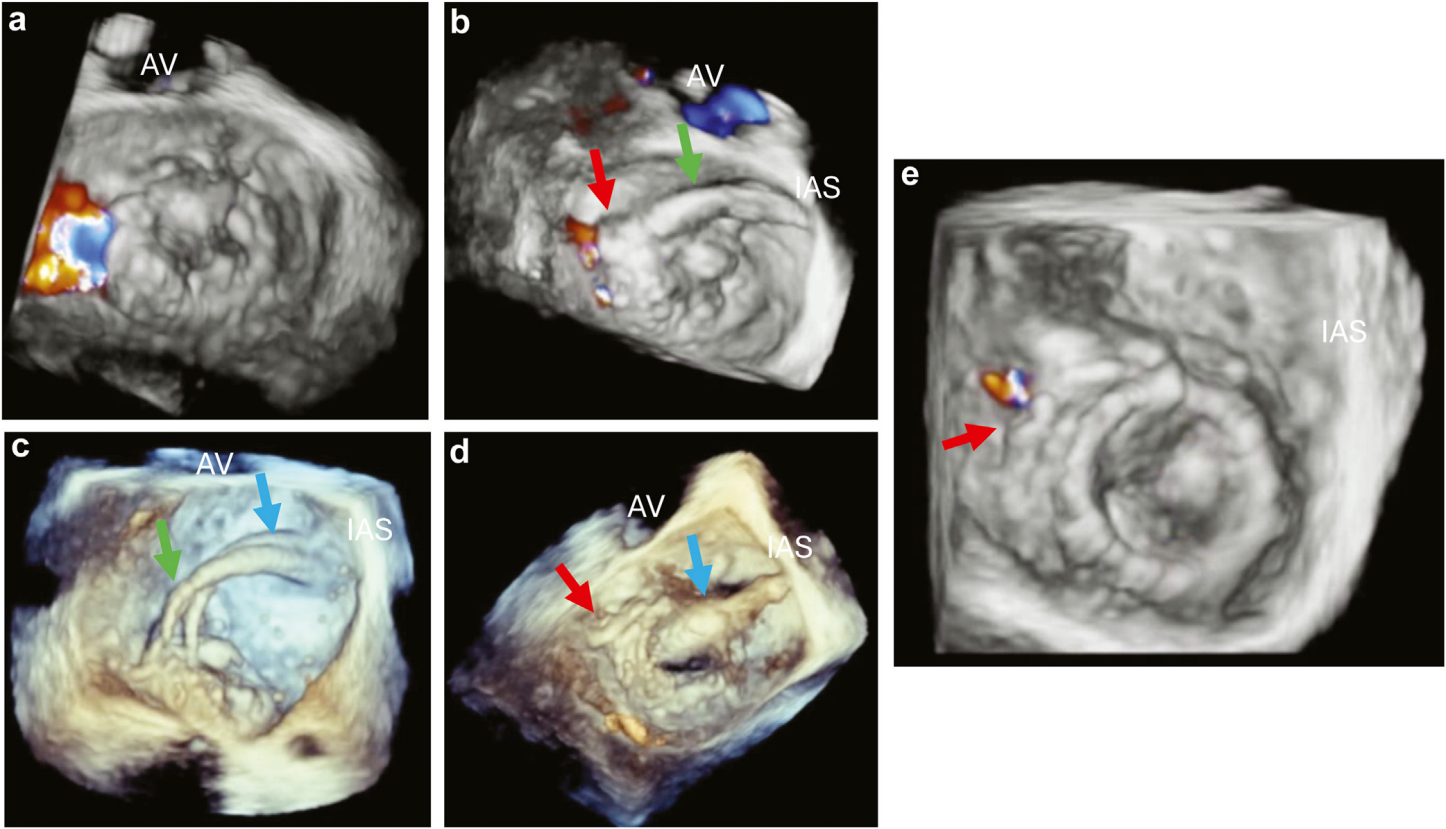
Patient with a bioprosthetic heart valve in the mitral position with severe valvular stenosis and severe paravalvular regurgitation. (a) Three-dimensional transesophageal echocardiographic *en face* view of the bioprosthetic valve in the mitral position during systole with severe paravalvular regurgitation located laterally. (b) Postplacement of a paravalvular leak closure device (red arrow). It is still attached to the delivery catheter (green arrow), which can be seen crossing the interatrial septum. Residual trace paravalvular regurgitation is still present. (c) Placement of the delivery catheter (blue arrow) across the prosthetic heart valve in preparation for the valve-in-valve procedure. (d) Postballoon inflation of the transcatheter aortic valve implantation (TAVI) valve within the bioprosthetic valve. The balloon (blue arrow) is still present across the TAVI valve-in-valve. The paravalvular leak closure device is also visualized (red arrow), but it is no longer attached to the delivery catheter. (e) Three-dimensional transesophageal echocardiographic *en face* image post-TAVI valve-in-valve demonstrating trivial paravalvular regurgitation from adjacent to the paravalvular closure device (red arrow). Abbreviations: AV, aortic valve; IAS, interatrial septum.
